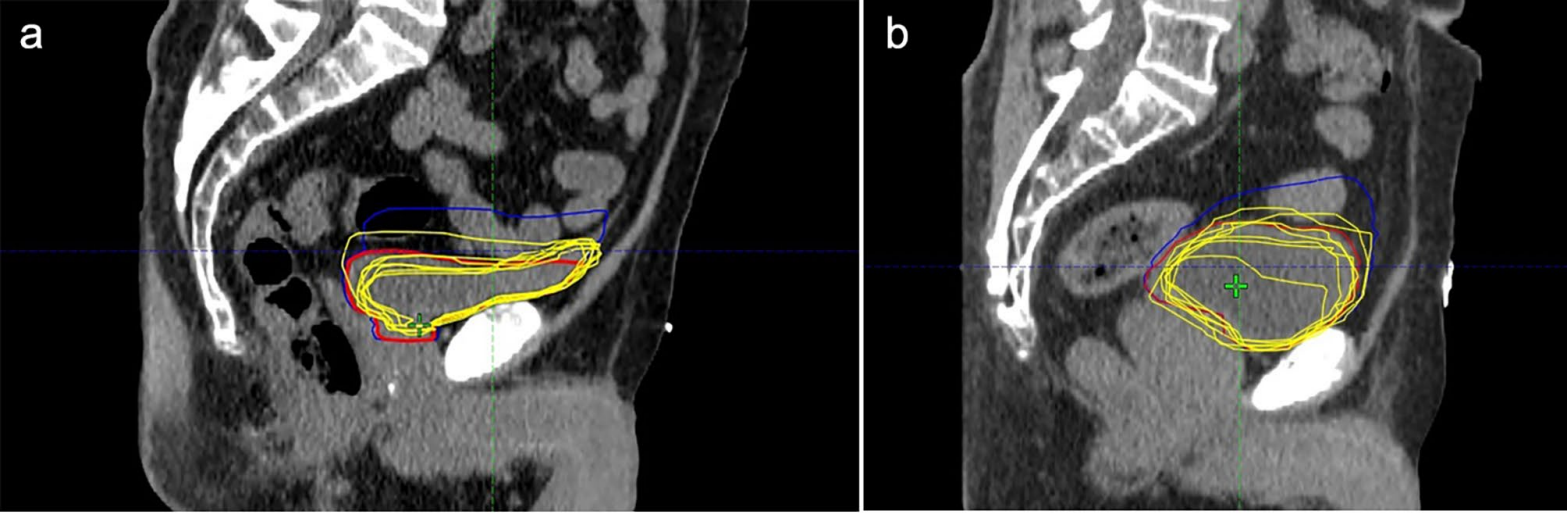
Sagittal CT slice for a patient from the BE (4a) and BF (4b) cohorts. Bladder volumes from treated fractions are shown in yellow, with the CTV from the first pCT in red and from the second pCT in blue. The larger variation in target size, shape and position with the BF protocol is evident
